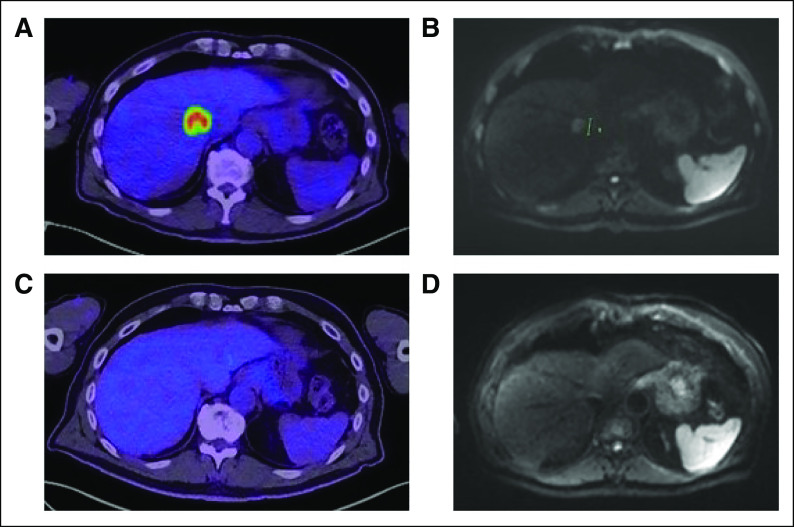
Response to olaparib after oligometastatic progression in liver. (A) PET scan and (B) MRI of liver after 5 months of maintenance pembrolizumab reveal progression in the sole remaining metastatic liver lesion. Olaparib was added, and 5 months later, a repeat (C) PET scan and (D) MRI demonstrate complete radiologic and metabolic response to therapy. MRI, magnetic resonance imaging PET, positron emission tomography.
